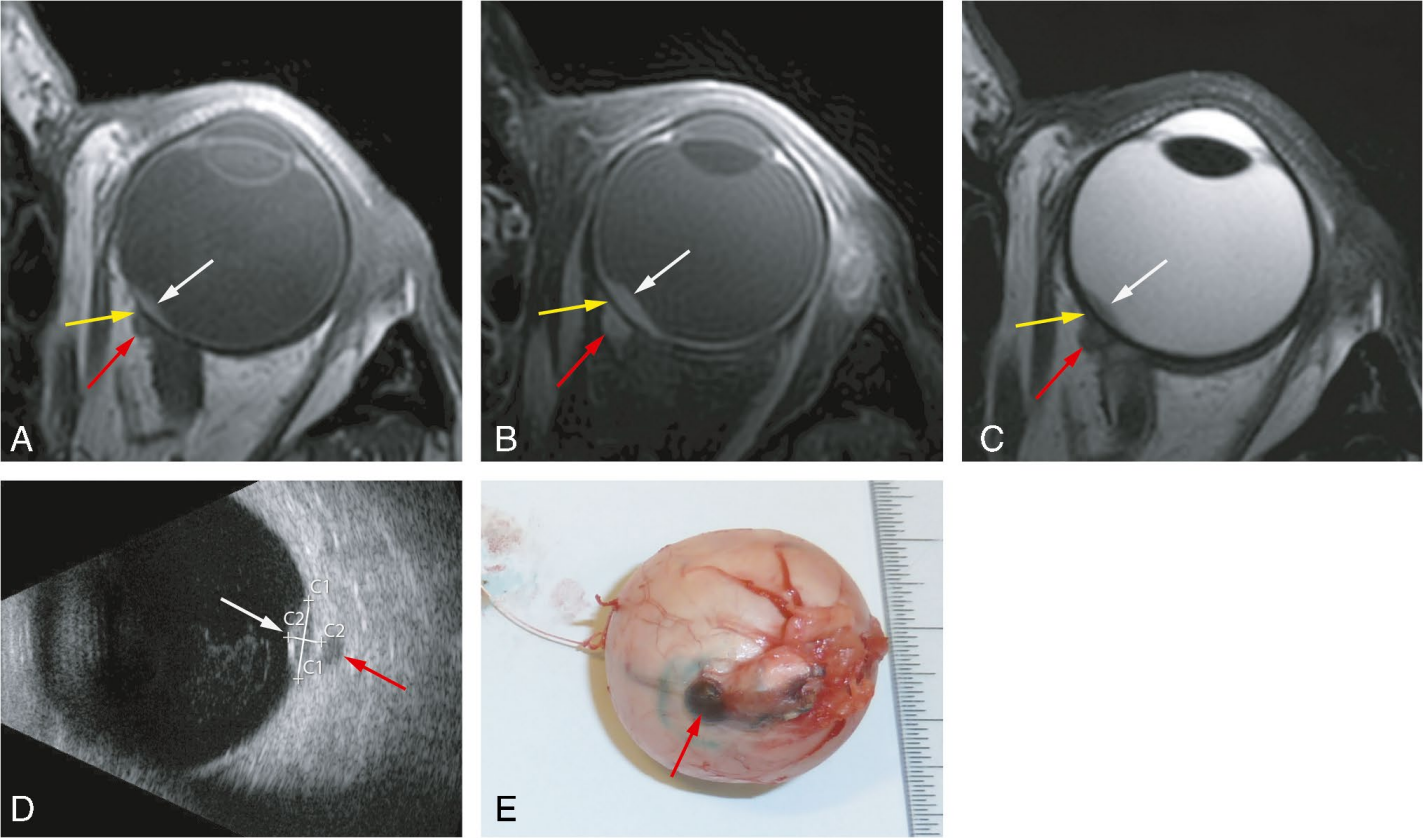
MR and grossing images of a uveal melanoma with extrascleral extension. A–C MRI with axials MS TSE T1-WI (A), contrast-enhanced T1-WI with fat signal suppression (B), and T2-WI (C). Small lentiform shape UM of the left eye (white arrow), with the extrascleral extension (red arrow) having, on all sequences, a signal intensity similar to its intraocular component. Notice the sclera, between the intra- and extraocular tumor components, with a normal aspect (yellow arrow). D B-scan ultrasound showing the small UM (white arrow) and also clearly the extrascleral extension (red arrow). E Enucleated eye at grossing with visible scleral and extrascleral extension (red arrow)
